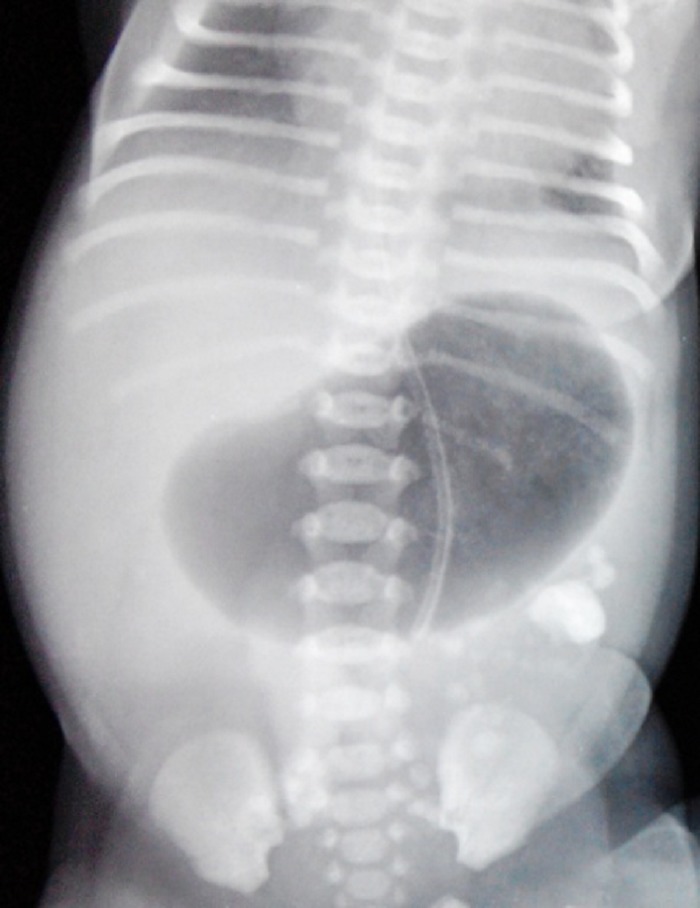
Figure 1: Abdominal radiograph showing single gastric bubble and calcification in left iliac fossa.
